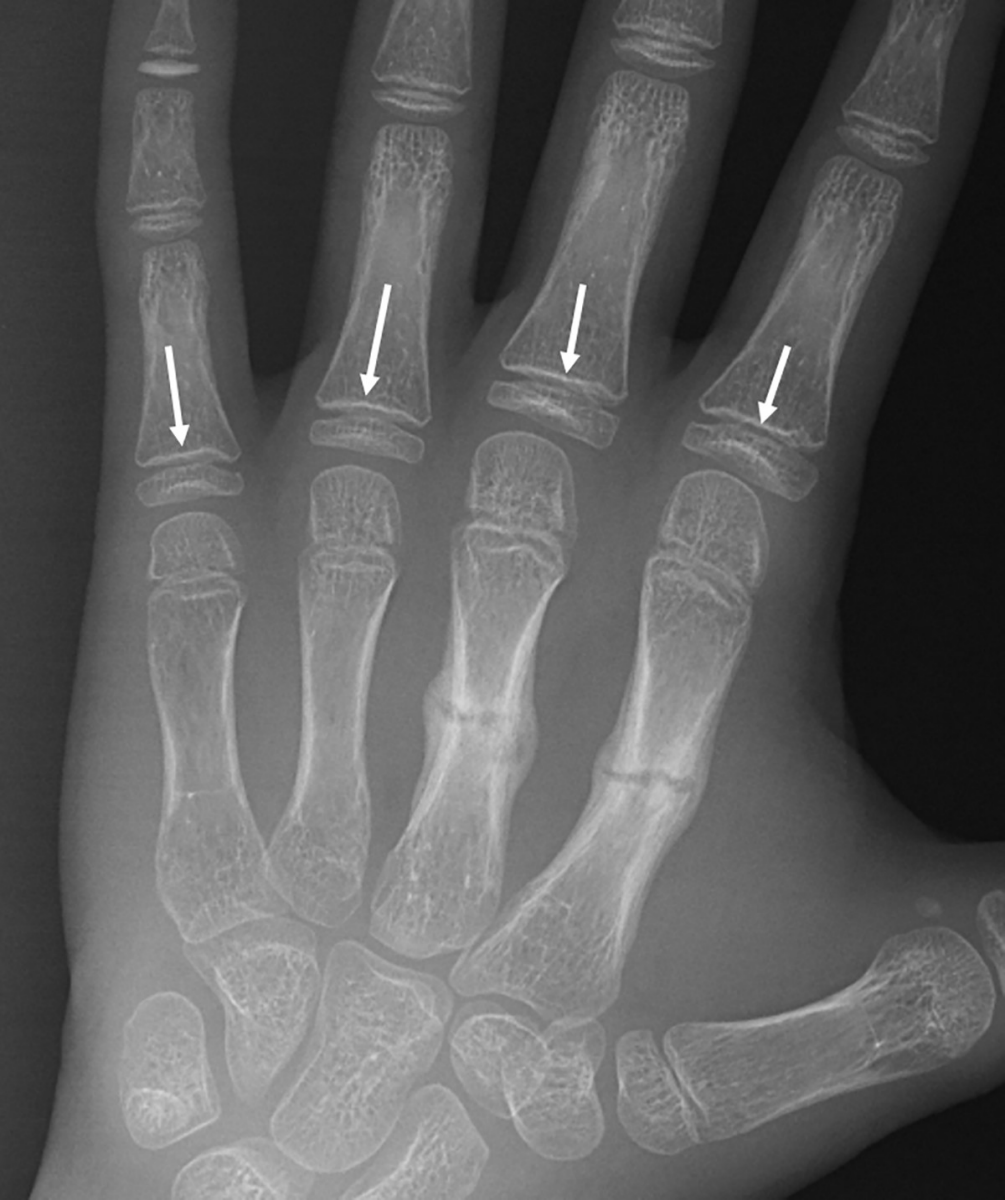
Radiograph of the hand in a 12-year-old boy with healing fractures to the 2^nd^ and 3^rd^ metacarpals, showing osteopenia due to inactivity. The zone of provisional calcification becomes more conspicuous, as its visualization is enhanced by the radiolucency in the metaphyseal side (arrows).
